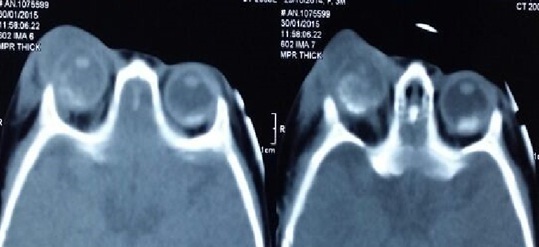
CT scan axial cuts of a bilateral orbital mass, optic nerve extension and orbital cellulitis of the right eye revealing a bilateral retinoblastoma with extra-ocular involvement
